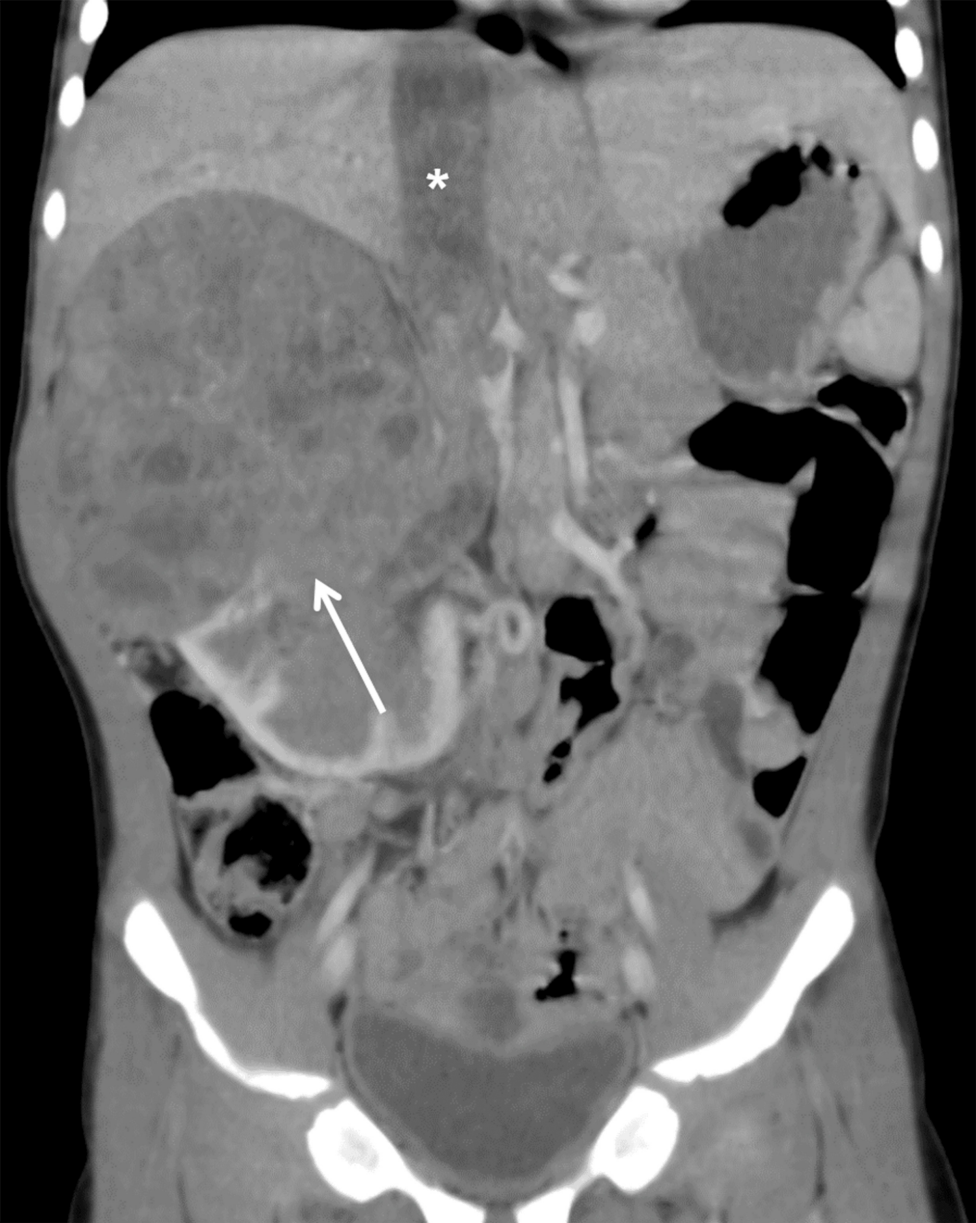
The coronal CT image shows WT in the right kidney (white arrow) and a tumor thrombus within the inferior vena cava (IVC) (*). CT: computed tomography; WT: Wilms tumor
